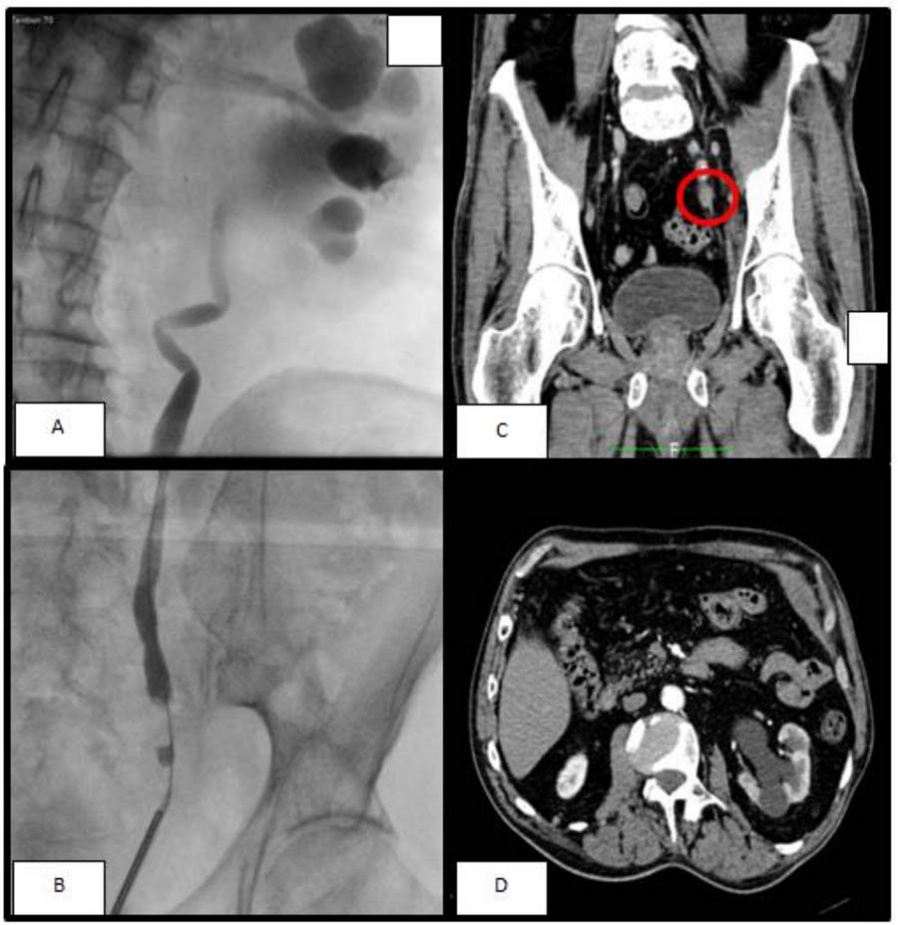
(A, B) The retrograde pyelography, performer before ureteroscopy, showed a minus contrast at the level of the pelvic ureter with an upstream hydroureteronephrosis; (C) left ureteral mass seen at arterious CT-scan; (D) the arterious CT-scan showed a hydronephrosis with corticalization of the calyxes.
